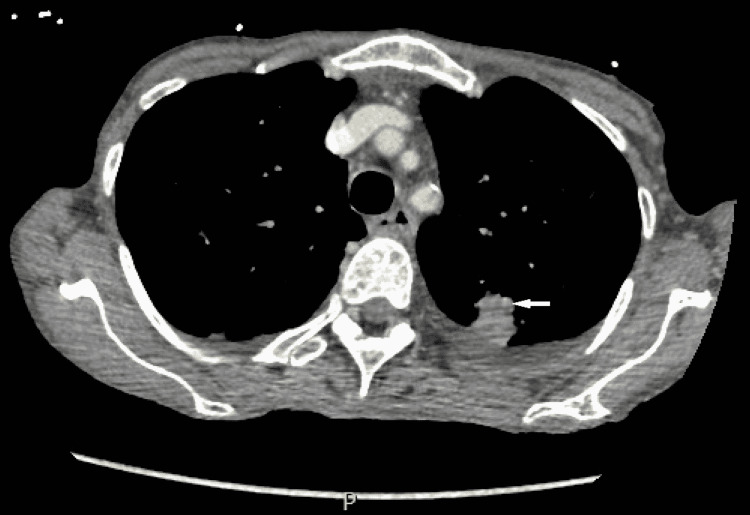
CT scan of the chest showing 21 mm (about 0.83 in) subpleural density in the posterior aspect of the left upper lobe. A white arrow shows subpleural density.
